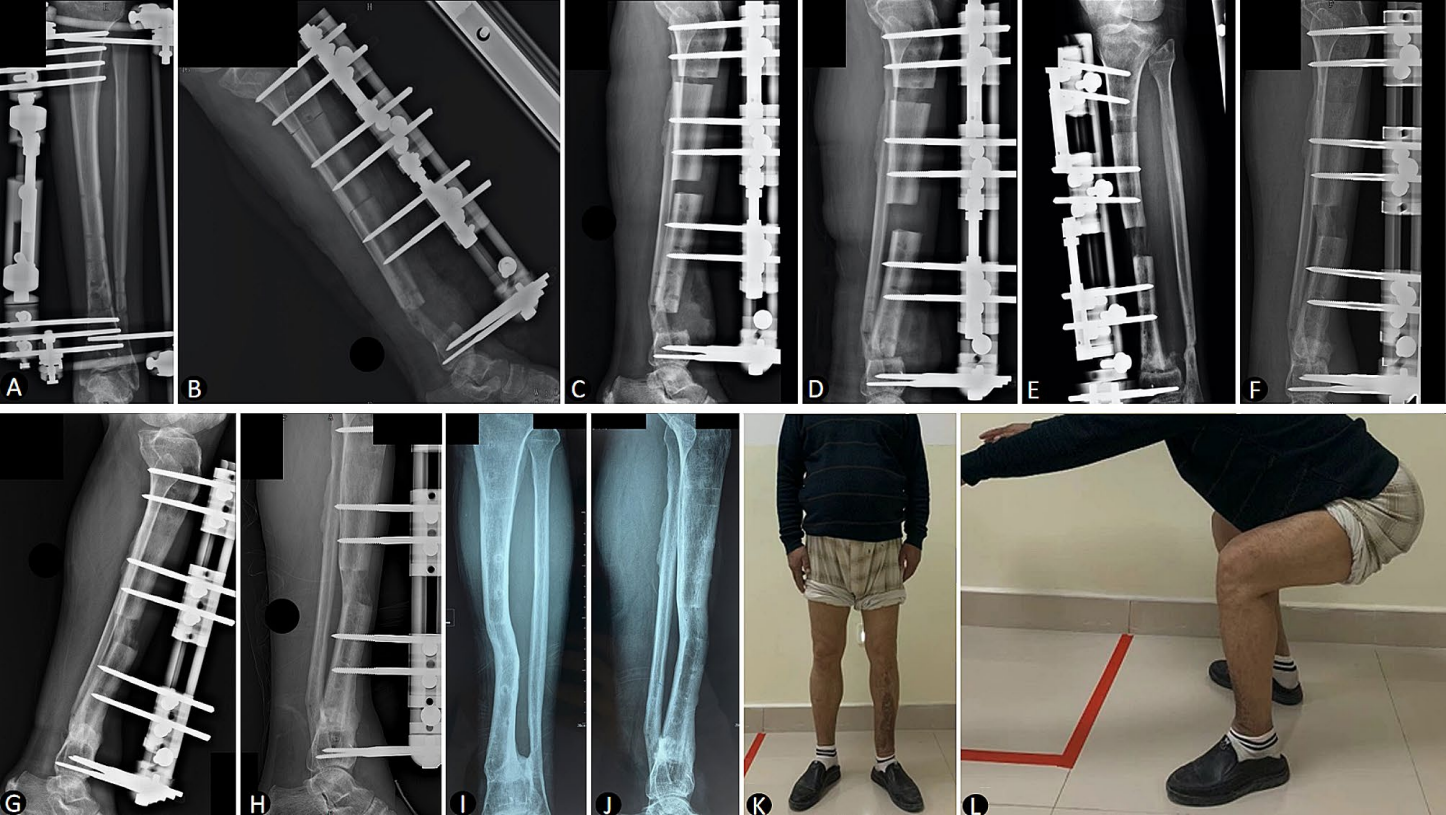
A A 52-year-old male patient with posttraumatic osteomyelitis of the left distal tibia. B Surgical removal of the infected bone and soft tissue resulted in a 6.3 cm defect, and beginning of trifocal bone transport. C, D,E A radiographic series delineating the trifocal bone transport phases, capturing the stepwise advancement of bone translocation. F, G,H Images taken at 1, 3, and 6 months post-docking, depicting progressive bone consolidation. I, J Anteroposterior and lateral radiographs demonstrating significant bone regeneration and proper alignment. K, L,M Functional assessment of the patient, exhibiting a restored range of motion and muscle strength during standing, squatting, and side bending movements, indicative of a successful trifocal bone transport process
